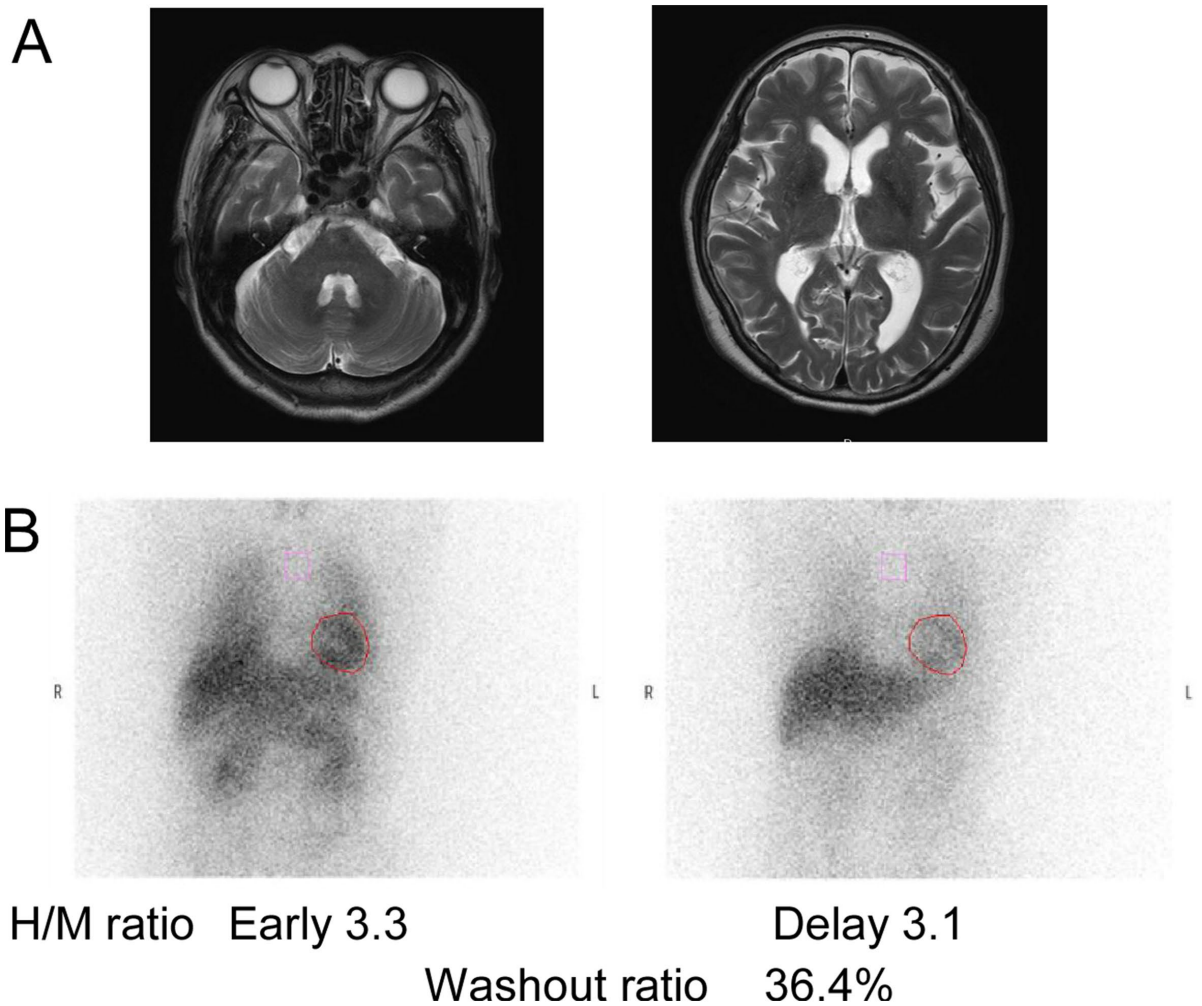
Imaging results of patients with *RFC1* repeat expansions. (A) MRI of the patient with AAGGG and ACAGG repeat expansions. (B)
